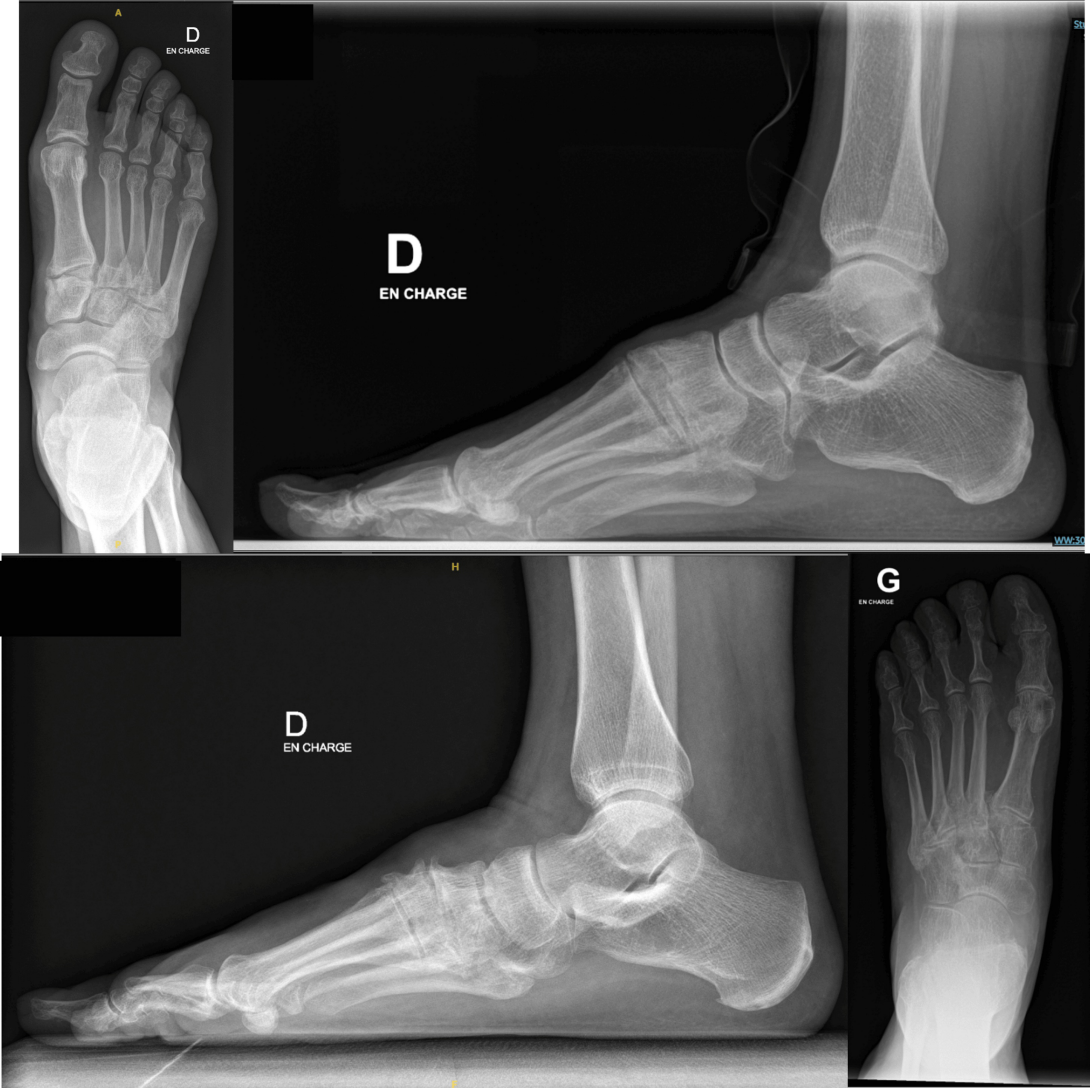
Examples of radiographs showing the Kellgren-Lawrence classification Upper left: Lawrence-Kellgren stage I; Upper right: Lawrence-Kellgren stage II; Lower left: Lawrence-Kellgren stage III; Lower right: Lawrence-Kellgren stage IV [14]
